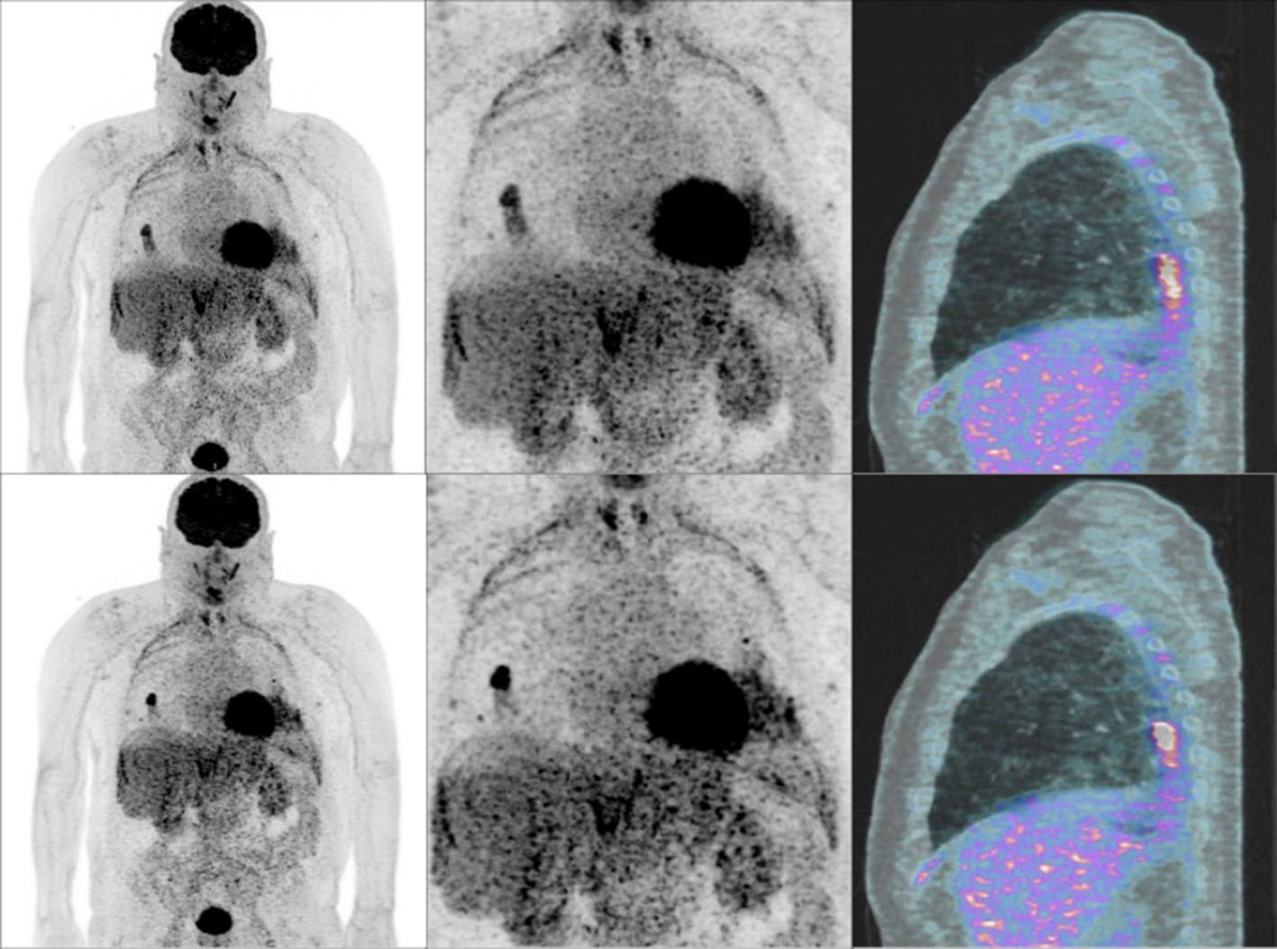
Free-breathing flow (first row) vs data-driven respiratory gating (second row) ^18^F-FDG PET/CT
